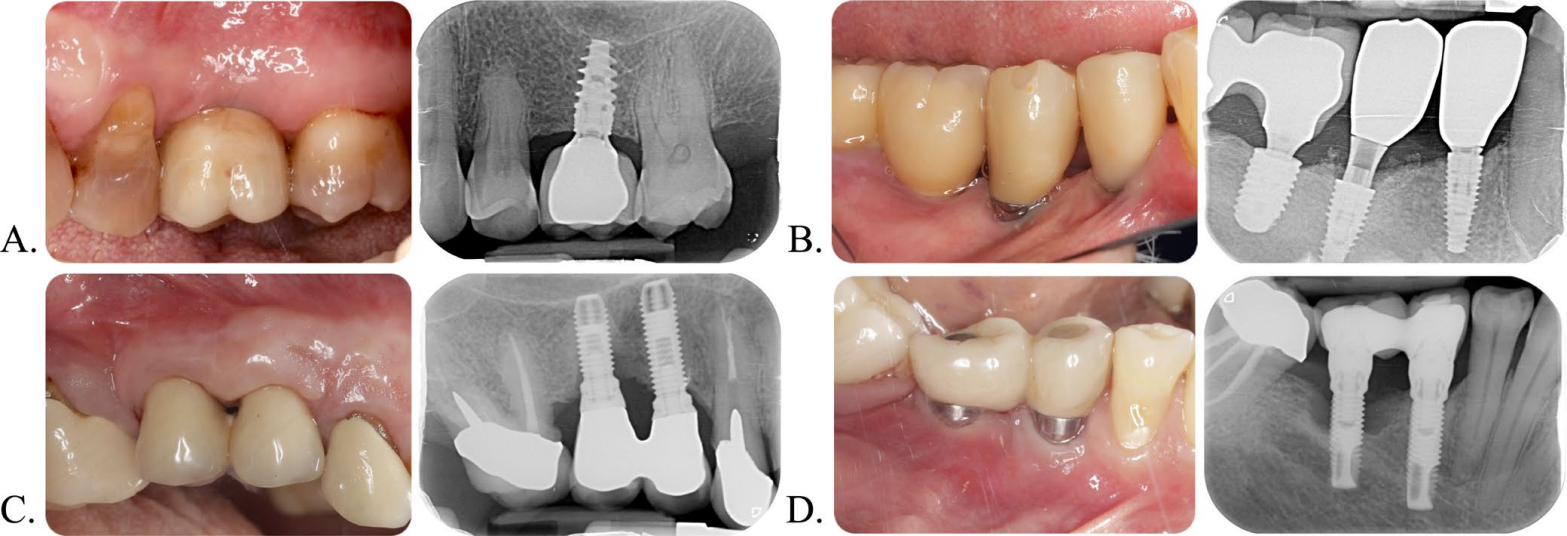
Clinical and radiographic examples of peri‐implant tissue conditions. (A) Healthy implant site without buccal soft‐tissue dehiscence. (B) Healthy implant site with buccal soft‐tissue dehiscence. (C) Implant affected by peri‐implantitis without buccal soft‐tissue dehiscence. (D) Implant affected by peri‐implantitis with buccal soft‐tissue dehiscence.
